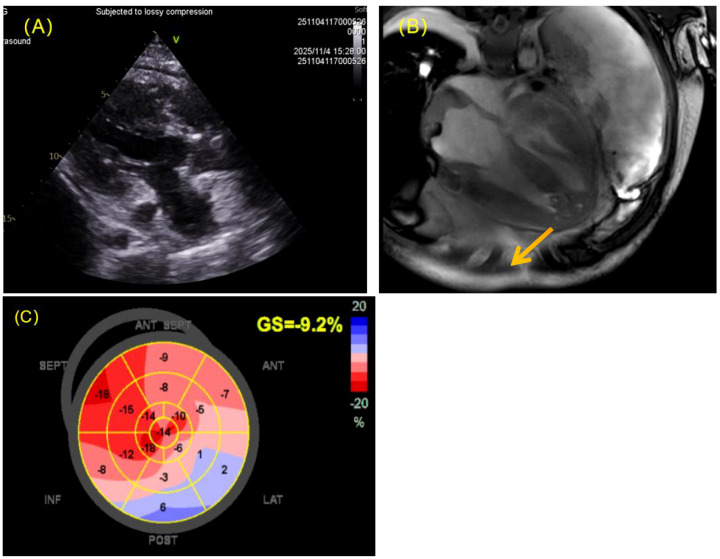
Cardiac imaging at presentation. (A) Transthoracic echocardiography reveals severe septal hypertrophy and right atrial enlargement. (B) CMR imaging shows diffuse myocardial hypertrophy and pericardial thickening. (C) Speckle-tracking echocardiography bull’s-eye plot demonstrates a markedly reduced global longitudinal strain (GLS) of −9.2%.
